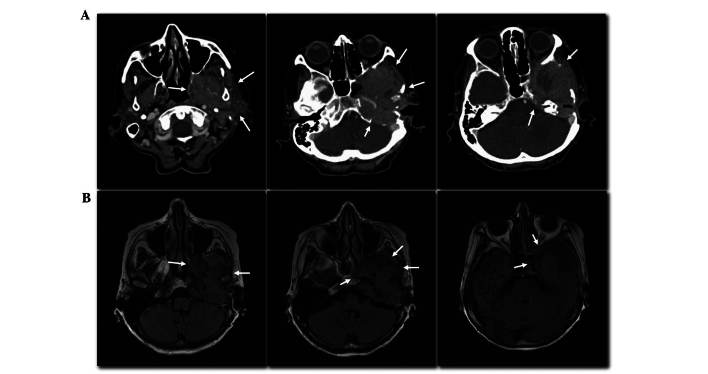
(A) Contrast-enhanced computed tomography (CT) imaging of the head shows a large soft tissue mass extending to the floor of the left middle cranial fossa with mild mass effect on the inferior temporal lobe. Destruction of the left mandibular condyle, left anterior temporal calvarium and the petrous of the left temporal bone are evident. The mass has eliminated the left jugular bulb and extends to the left cavernous sinus and the lateral wall of the orbit. (B) Magnetic resonance imaging (MRI) imaging verifies the involvement of the left cavernous sinus and the dura along the sphenoid and temporal bones. The left carotid artery shows significant narrowing suggesting circumferential involvement. The left pterygoid muscle and temporomandibular joint are also involved in the malignant process. The images suggest intraorbital extension of the tumor.
